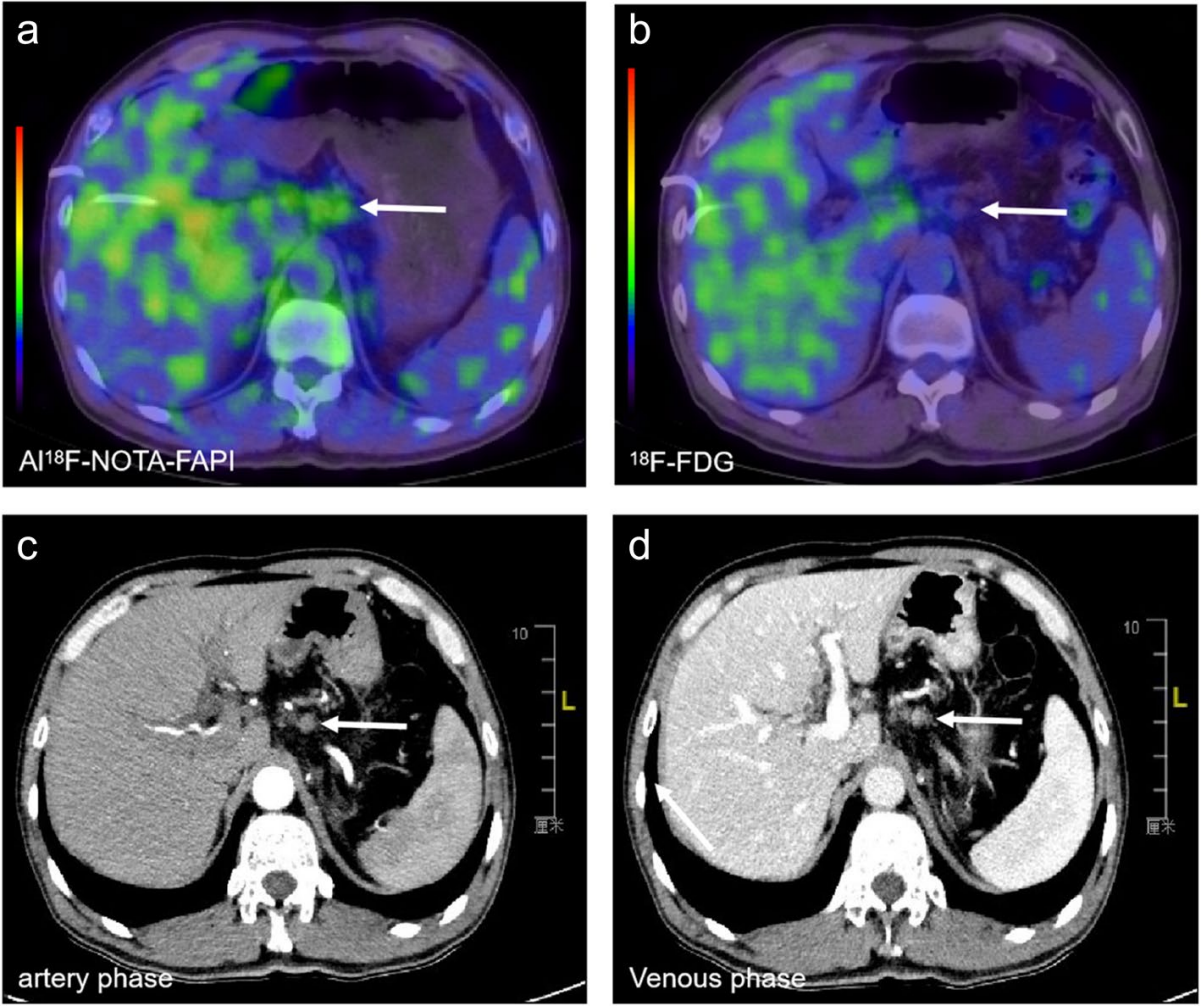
An evaluation of a patient with a local PDAC. Al^18^F-NOTA-FAPI-04 PET/CT showed increased FAPI uptake in lymph nodes (a, white arrow, SUV_max-15min_=4.8), which all showed no uptake in ^18^F-FDG PET/CT (b, white arrow). Meanwhile, the contrast-enhanced CT showed little enhancement in both arteries (c, white arrow) or the venous phase (d, white arrow). Thus, the N stage was upstaging to the IIB
